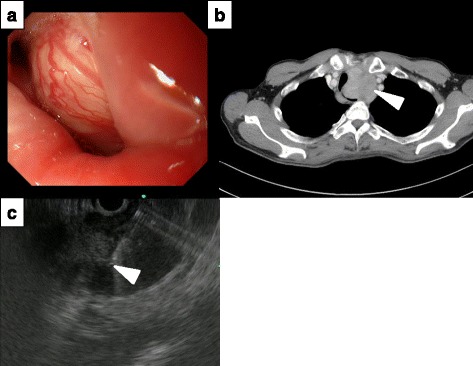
The examination image before the operation. a Endoscopy revealed an approximately 5.0 cm esophageal submucosal tumor, which was located 25 cm from the incisors. It occupied the majority of the esophageal lumen. b Computed tomography revealed a large isodense tumor of the esophageal wall in the upper mediastinal space (*white arrow*). c Endoscopic ultrasonography showed a large tumor (*white arrow*) of low echogenicity in the submucosa layer
